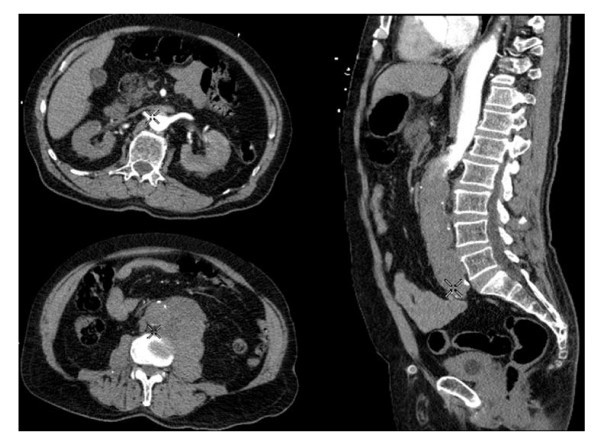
CT aortography. An 11.5 × 9 × 6 cm solid mass lying on the left side of the mid-abdominal aorta is shown. Contrast was seen extending to the level of the mass. Coeliac axis, superior mesenteric and renal arteries were filled but no opacification was present through the distal aorta and iliac arteries. Some contrast was seen in distal external iliac arteries, presumably arising from collateral vessels. Both kidneys did not enhance well, suggesting vascular compromise in both.
